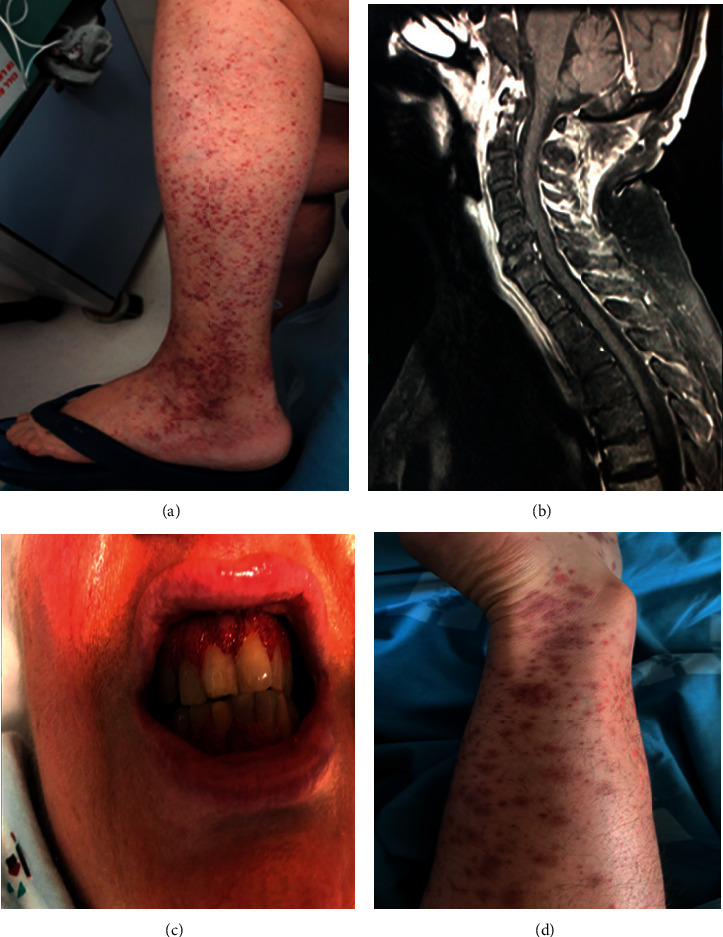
Clinical presentation of patients with autoimmune disease following SARS-CoV-2 infection. (a) Lower limb purpuric lesions in a patient with Henoch–Schönlein purpura. (b) MRI scan in T-1 fat-suppressed sagittal view, showing enhancement and edema in the frontal and posterior cervical paravertebral soft tissues, as well as C2 osteitis, in a patient with a polymyalgia rheumatica-like disease. (c) “Strawberry gingivitis” as a presentation of granulomatosis with polyangiitis. (d) Left lower limb petechial eruption in a patient with cryoglobulinemic vasculitis.
